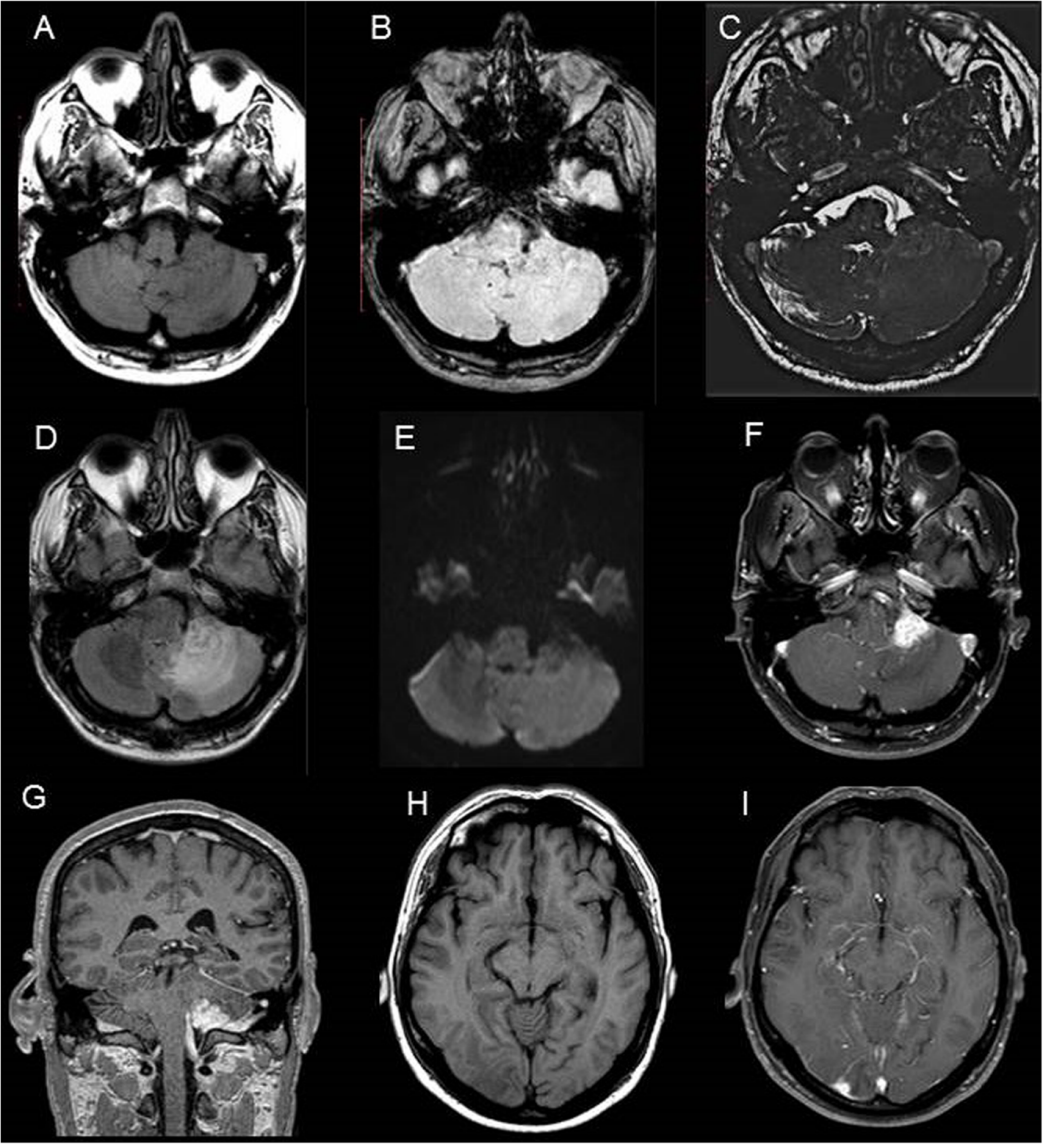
Neuroimaging findings in the patient with Rosai-Dorfman disease demonstrating extra-axial nodular image located in the left cerebellopontine angle cistern with isosignal in T1 and low signal in T2 / FLAIR with homogeneous contrast enhancement, surrounded by cerebellar edema, with dural tail and no restricted diffusion (A, B to G). In H and I there is a small extra-axial image with the same characteristics described above in the right temporal region
